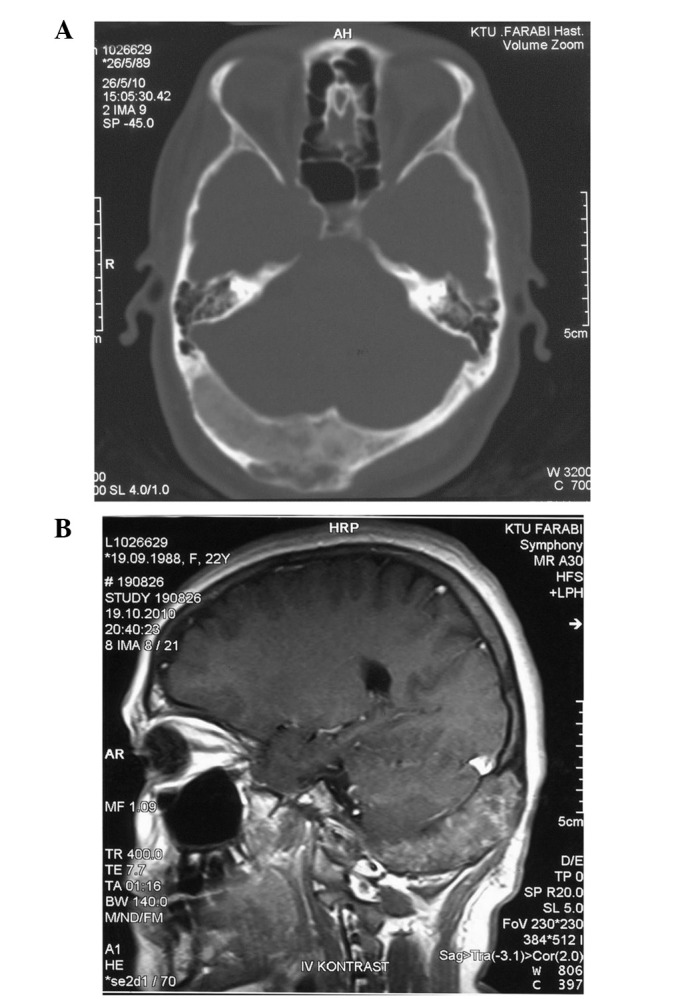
(A) Brain computed tomography revealing a ground-glass appearance within lytic areas, 2.5×6 cm. (B) Cranial magnetic resonance image showing a mass, of equal intensity with the muscle tissue, of 2.5×6 cm on the right side of the occipital bone.
